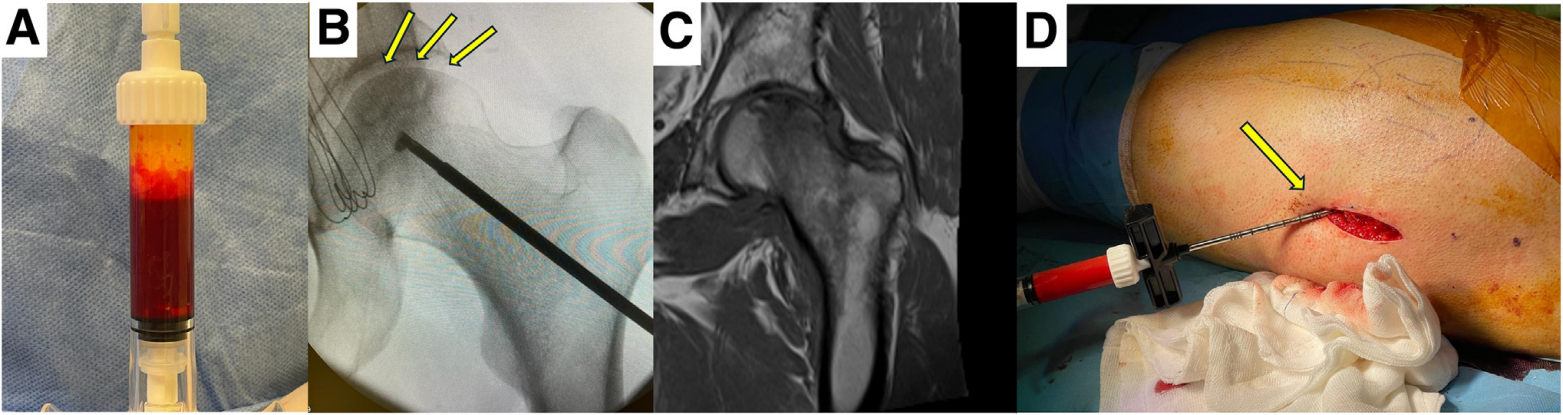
(A) The bone marrow aspiration concentrate (BMAC) and stromal vascular fraction (SVF) aspirates are mixed. (B, C) The decompression procedure is achieved through a guidewire and retro-drilling to decompress and debride the osteonecrotic and edematous region, according to the intraoperative fluoroscopic and magnetic resonance imaging views (arrows). (D) A thin cannula is then placed at the entrance of the decompression tunnel (arrow), and the previously obtained crushed autografts together with the BMAC and SVF mixture are gently injected into the debrided area. The drill entrance is then sealed with the intact fragment of the cortical graft (left hip, patient supine, caudad to cephalad from left to right).
